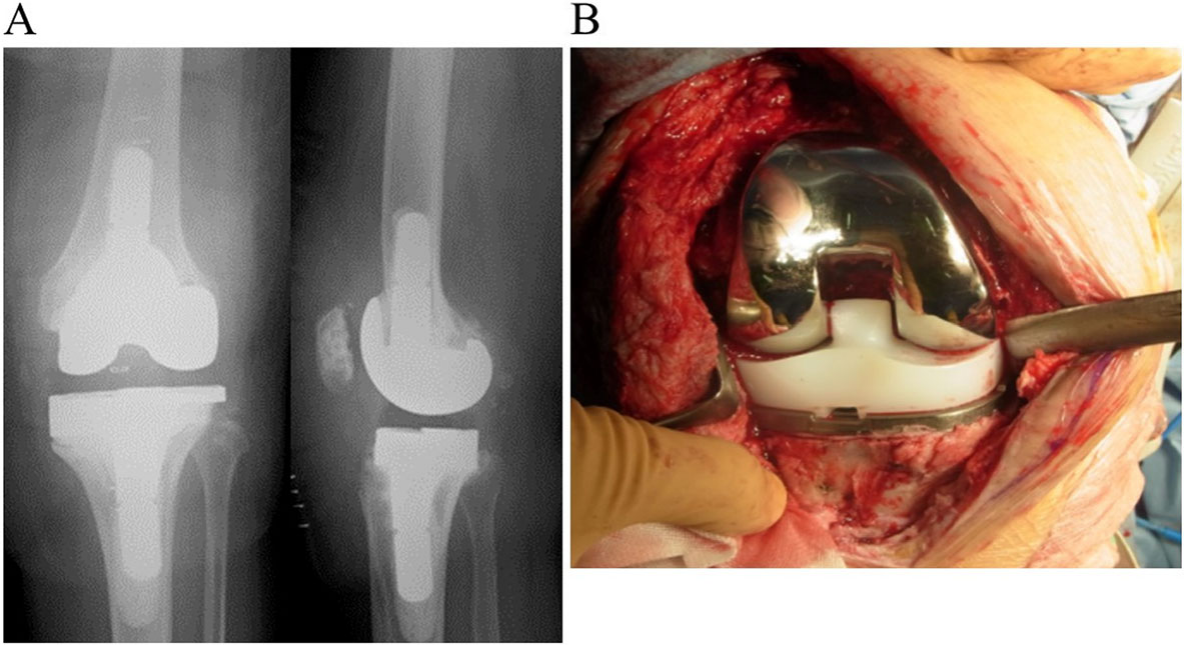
Second-stage surgery: revision total knee arthroplasty (TKA). A Radiograph at final follow-up. Revision TKA was completed without implant loosening. B Macroscopic image of TKA prosthesis
